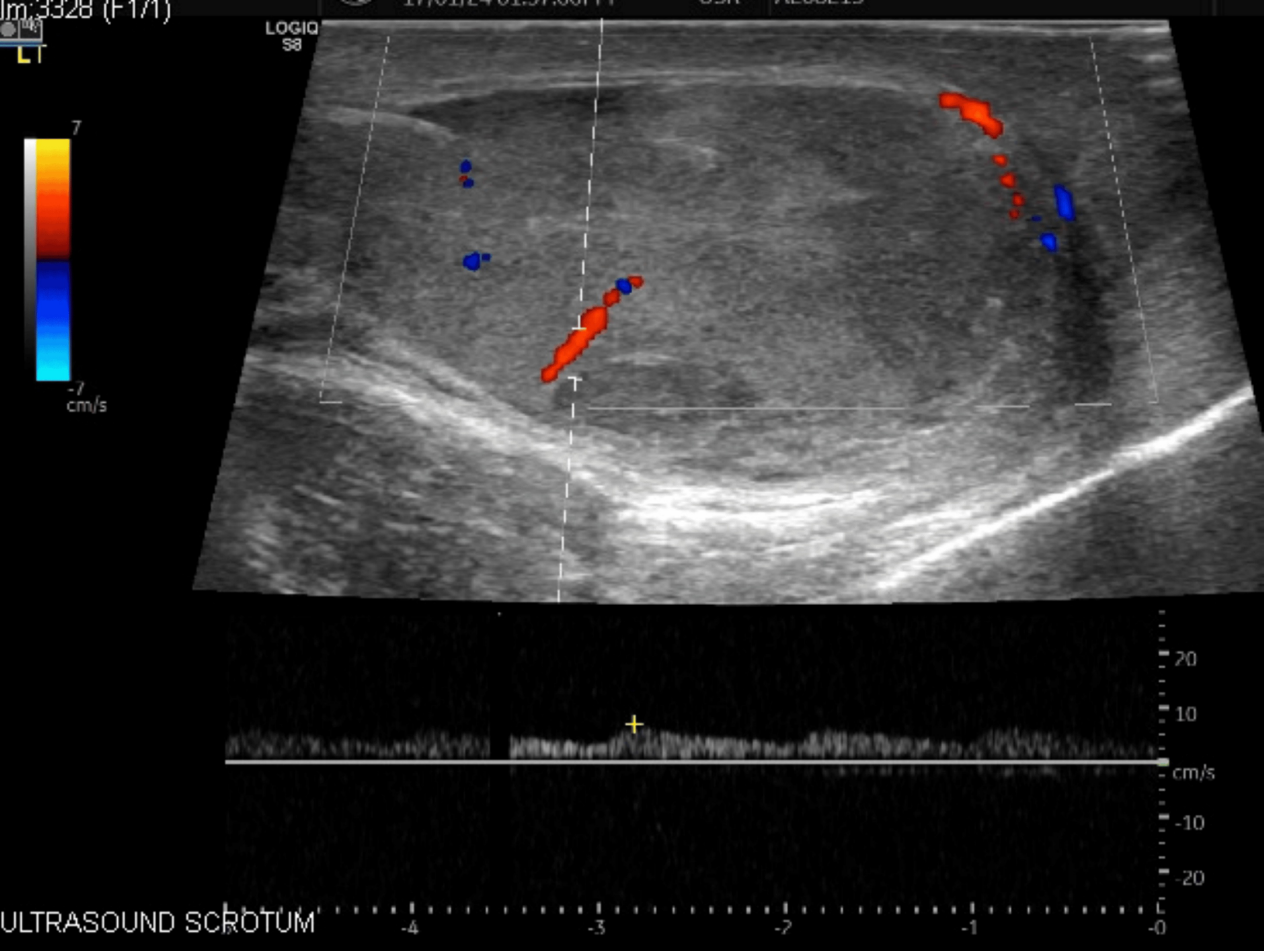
Postoperative ultrasound image showing the left testis with preserved vascularity.
